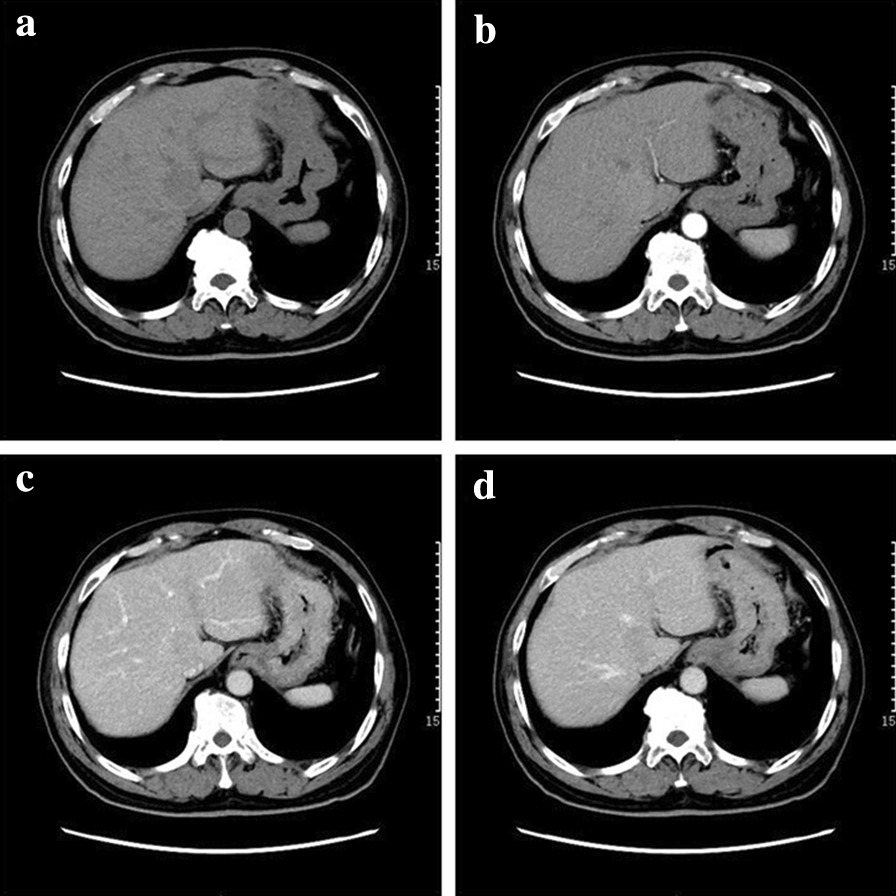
A 64-year-old male patient with inflammatory pseudotumor-like follicular dendritic cell sarcoma of the liver. a Plain computed tomography scan showing a round, slightly hypodense lesion with homogeneous density and no obvious necrosis, calcification, or bleeding in the caudate lobe of the liver. b Slight enhancement in the arterial phase. c and d Decreased enhancement in the portal and delayed phases, respectively
